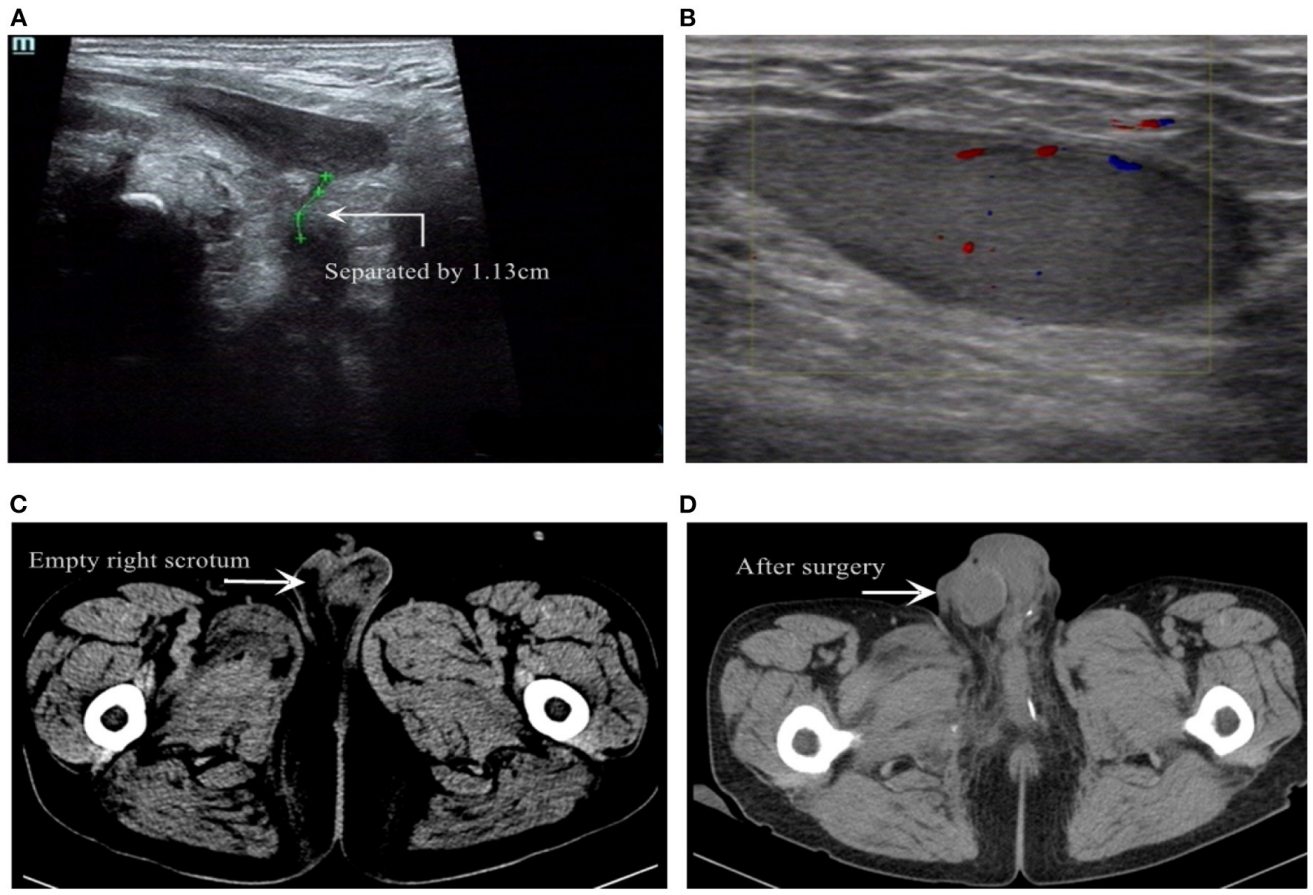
(A) Transpelvic ultrasound examination revealed membranous urethral rupture. (B) Color Doppler US image of the inguinal mass revealed a intact shape of testicle with blood supply. (C) Pelvic CT showed the swollen scrotum and the empty right scrotum. (D) Re-examination of pelvic CT after surgery.
